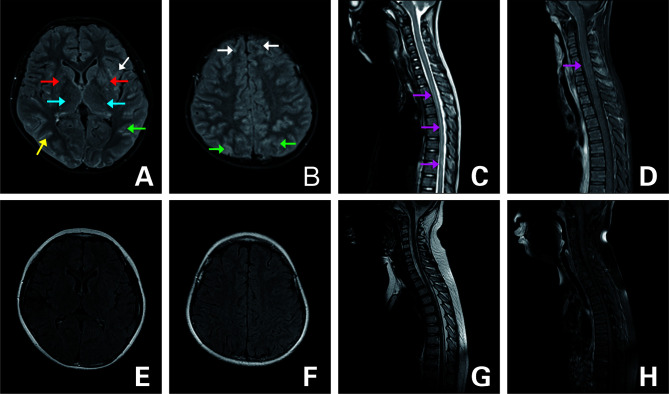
FLAIR MRI of the brain and spine (A–D) upon admission and (E–H) 3 months later. (A, B) T2-hyperintense lesions in bilateral frontal (white arrow), parietal (green arrow), occipital (yellow arrow) cortex and white matter, basal ganglia (red arrow), thalamus (blue arrow), and diffuse enhancement. (C) Lesion in the T1-L1 (purple arrow). (D) Strip enhancement of spinal meninges (purple arrow). (E–G) Follow-up images of improved T2 lesions. (H) Follow-up image of the enhancement after it disappeared.
